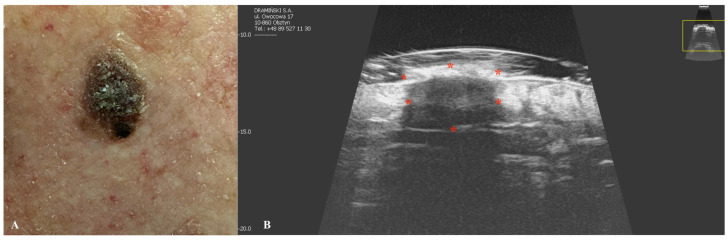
Macroscopic view of malignant melanoma (A) with a corresponding ultrasonographic image (B). Stars indicate tumor margins. The yellow box indicates the area covered by the ultrasonographic field of view.
